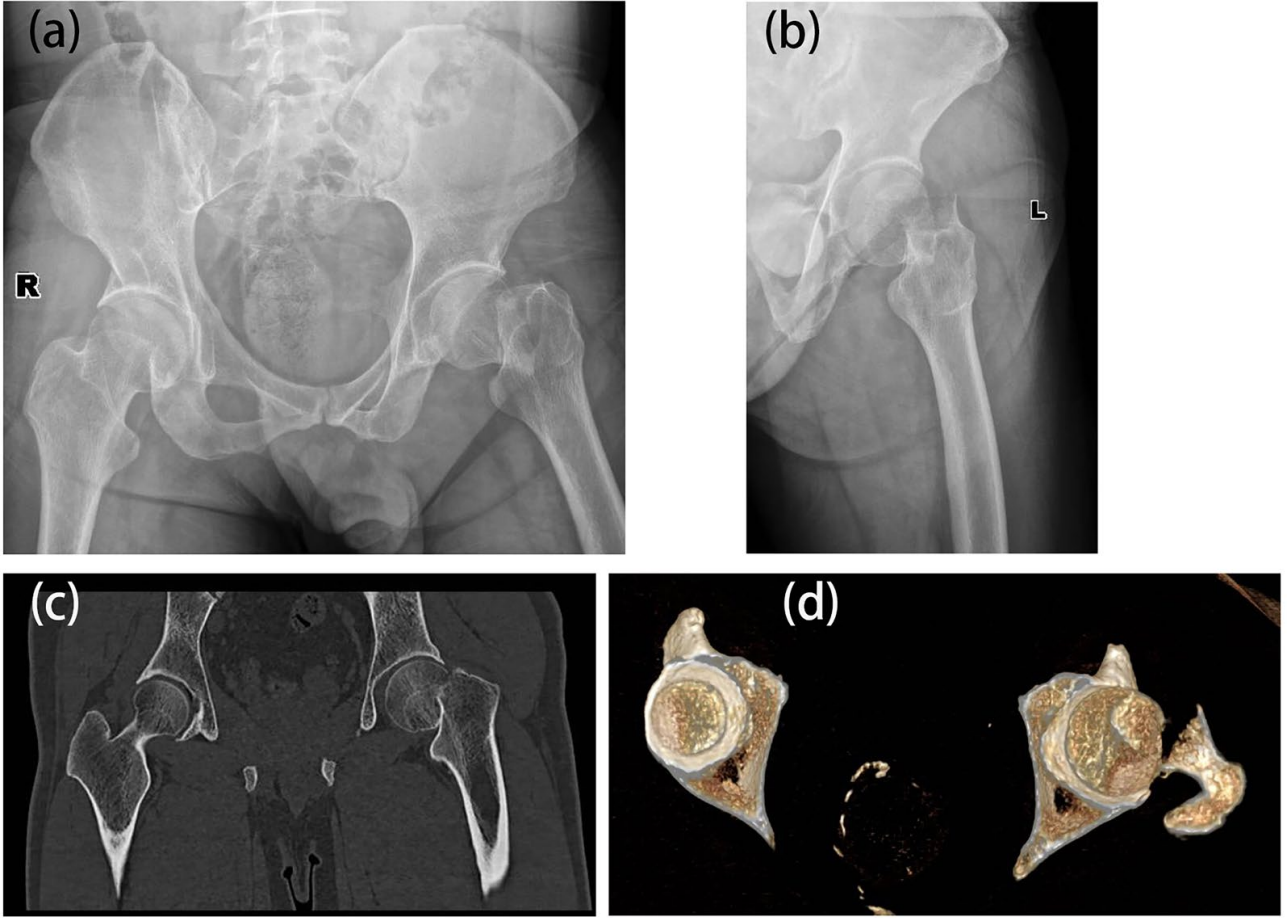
Preoperative X-ray and CT scan showing left femoral neck fracture (Garden IV type): a hip joint in an upright position; b left hip joint lateral position; c-d preoperative CT revealing complete displacement of the left femoral neck fracture
